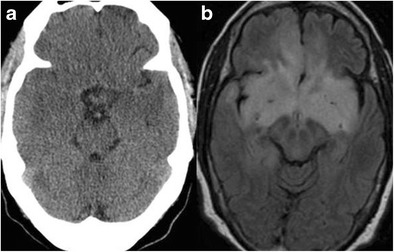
A 42-year-old female with new onset seizures. On noncontrast CT (a), only mild mass effect is noted on the Sylvian fissure and subtle symmetric hypodensity in the temporal lobes. On MR (b), the abnormality is clearly visualized as bilateral temporal and insular FLAIR hyperintensity, crossing midline along the anterior commissure and also extending to the frontal lobe. The lesion did not enhance and biopsy confirmed the diagnosis of low-grade glioma
